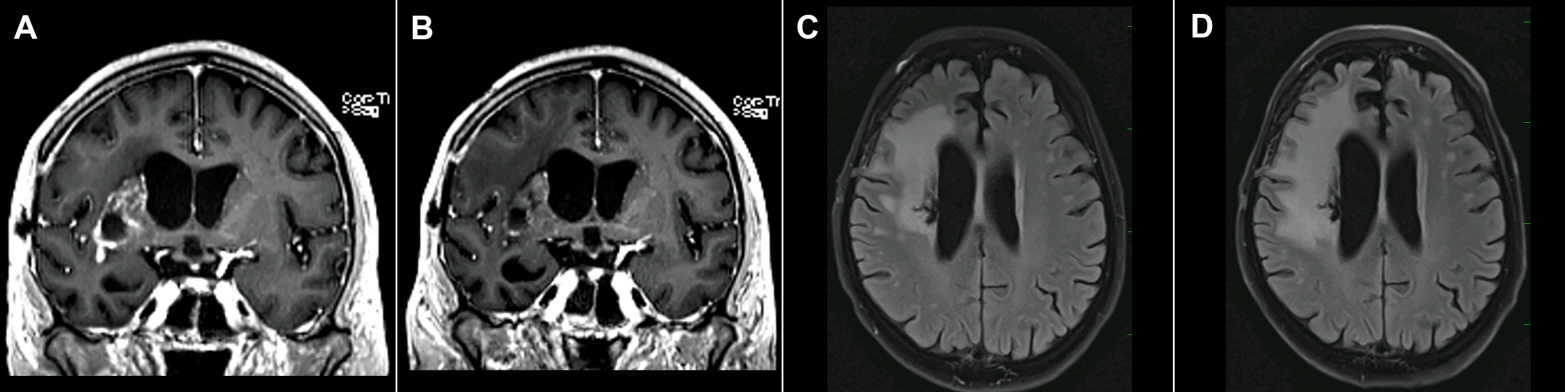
59-year-old female. (A) April 2019, 3 Tesla scanner “bevacizumab-like” TTFields effect: T1-weighted postcontrast gradient echo postoperative image with enhancing residual GBM in the region of the basal ganglia on the right side. (B) July 2019, 3 Tesla scanner: T1-weighted postcontrast gradient echo image (identical scanner, sequence parameters, type and dose of the contrast agent [Gadovist 6 ml]) shows a decrease in postcontrast enhancement. However, the neurological status of the patient progressed. (C) April 2019, 3 Tesla scanner, FLAIR image and (D) July 2019, 3 Tesla FLAIR image show edema progression at the time when enhancement decreased.
